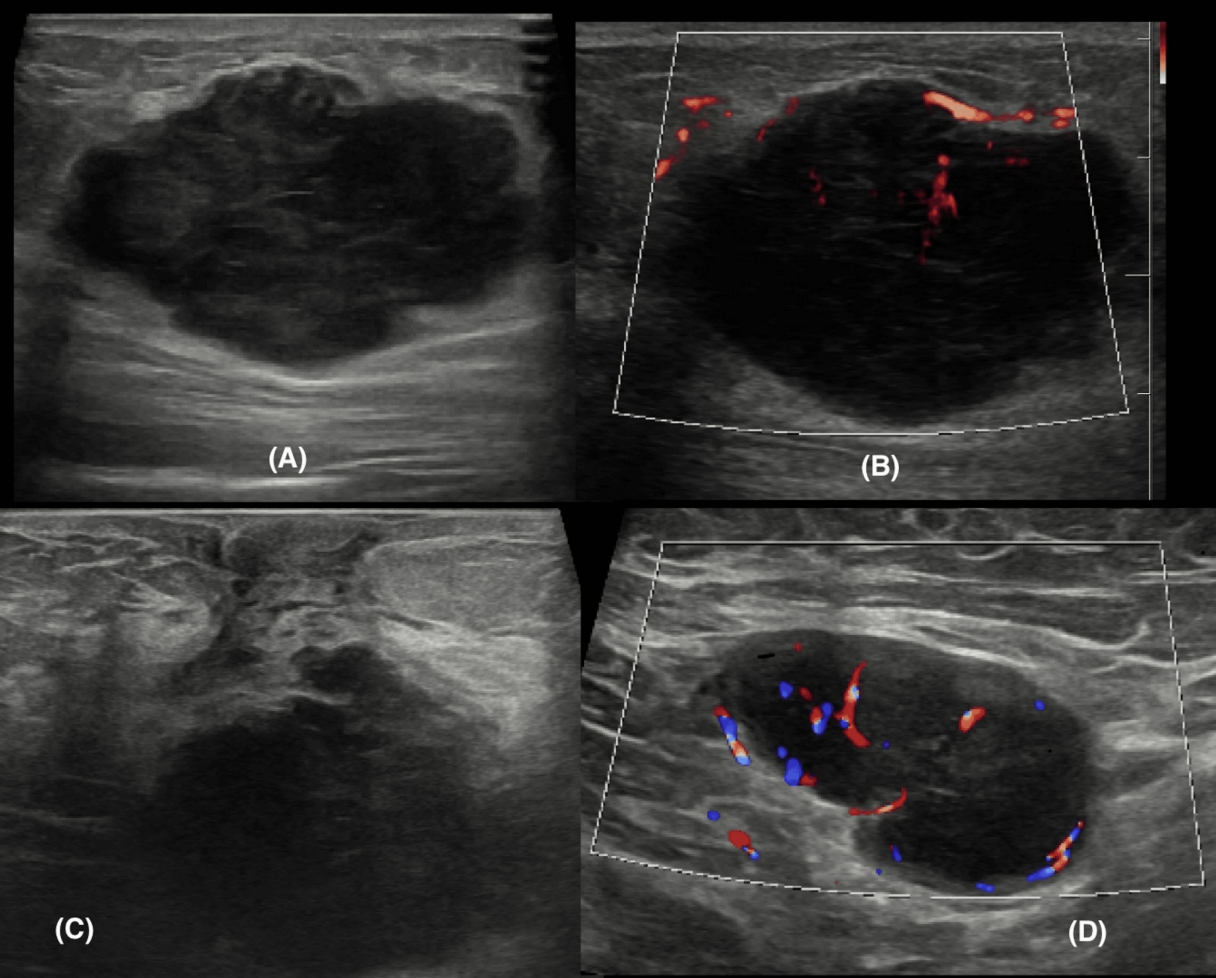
Ultrasound (A) shows a circumscribed hypoechoic irregular mass with internal vascularity on Doppler (B). An abnormal axillary lymph node with cortical thickening and increased vascularity is also noted.
